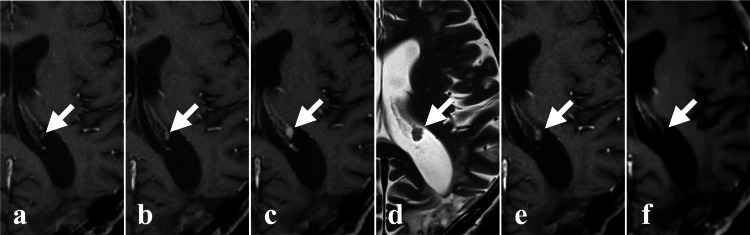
Time course of the choroid plexus lesion in the left lateral ventricle on axial MRI. (a) Lesion is not evident (indicated by the white arrow). (b) Slightly increased enhancement of the lesion 1 month after (a) MRI (indicated by the white arrow). (c) Further increase in the lesion 1 month after (b) MRI (indicated by the white arrow). (d) Further increase in the lesion on T2WI 2 months after (c) MRI (indicated by the white arrow). MRI showing the maximum lesion 2 weeks before PZP starts. (e) Shrinkage of the lesion 3 weeks after (d) MRI (indicated by the white arrow). MRI showing the decreased lesion 6 days after PZP start. (f) Further shrinkage of the lesion 15 weeks after (e) MRI (indicated by the white arrow). MRI showing complete response (CR) of the lesion 16 weeks after PZP start. T2WI: T2-weighted image; CR: Complete response; PZP: Pazopanib.
